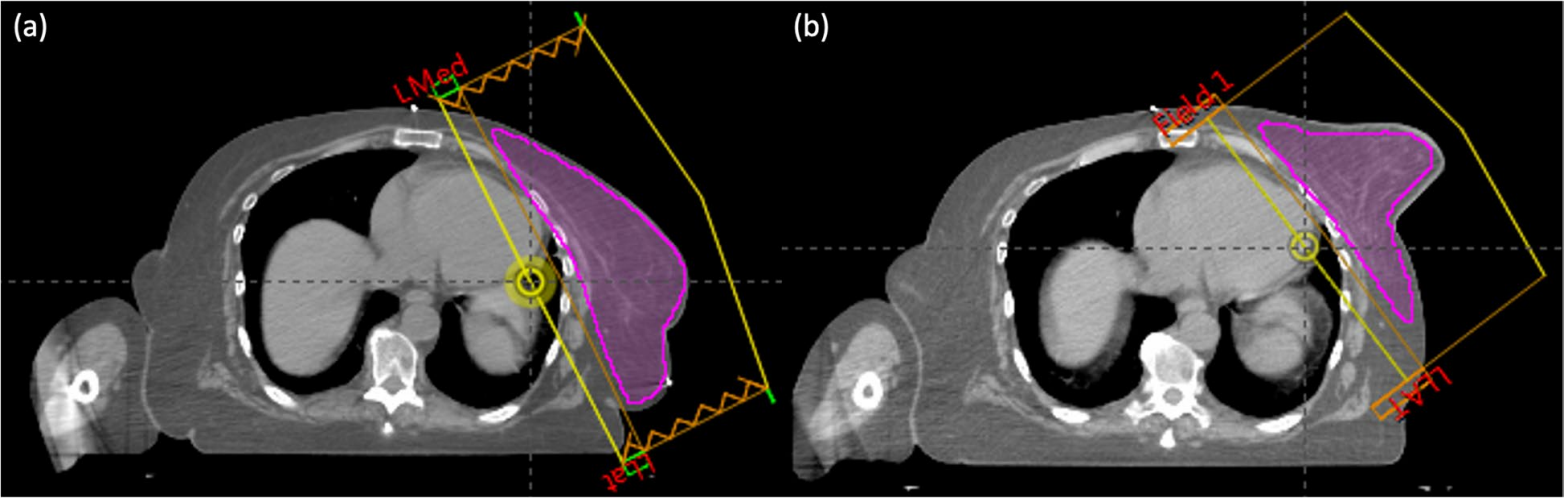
Axial CT images of the patient shown in Fig. 1 without CARA (a) and with CARA (b). The tangential beams and target volume are shown
